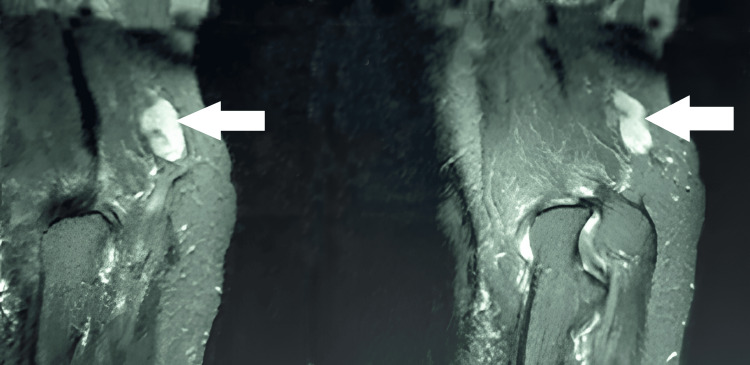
Magnetic resonance imaging (sagittal view) reveals a mass arising from the left ulnar nerve at the elbow
